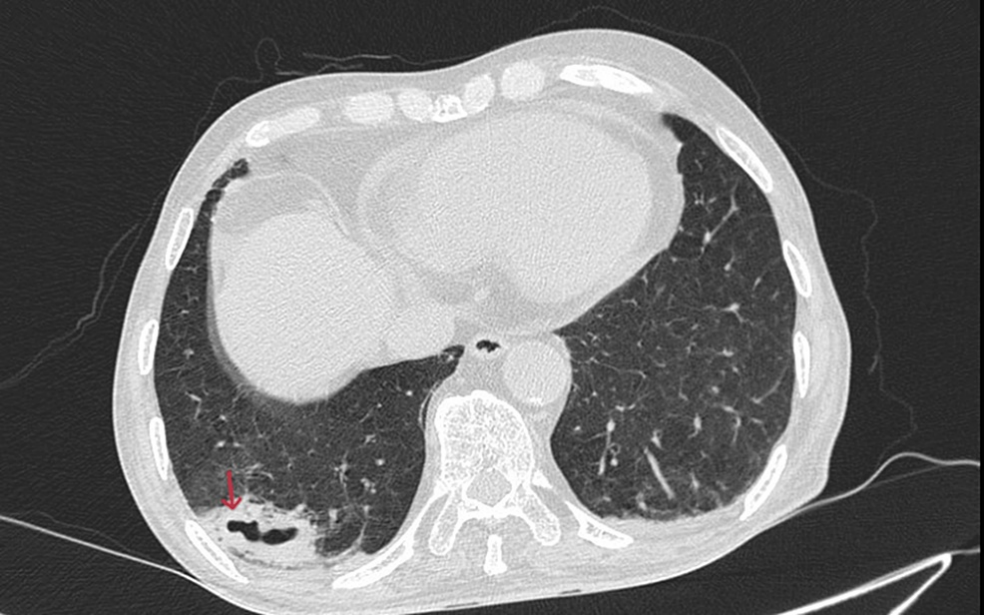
Axial CT lung window showing consolidation with cavitary lesions in the lower lobe of the right lung (red arrow). CT, computed tomography
